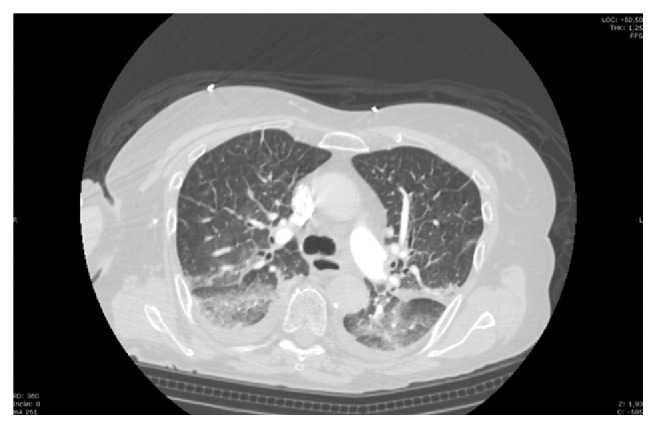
Lung CT scan of the lung.
